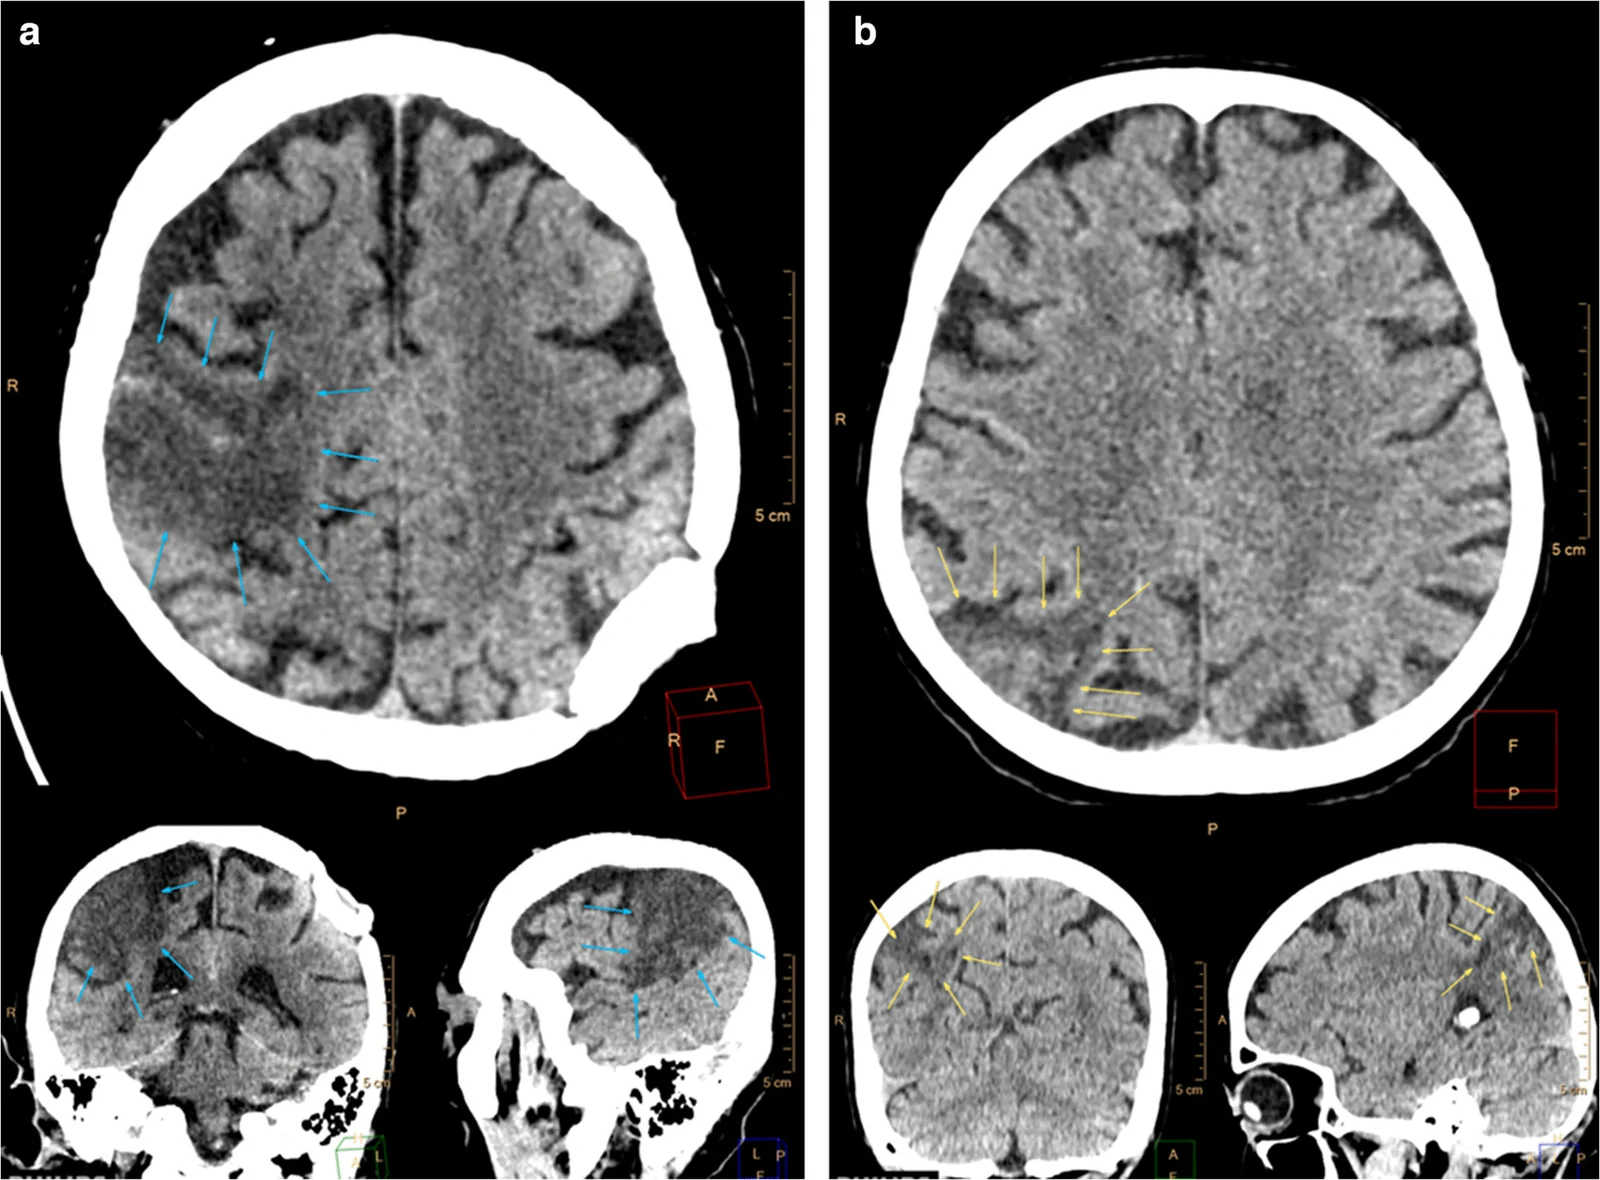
a, b Multiplanar CT reformats of a voluminous ill-defined cortical-subcortical hypodensity in the right frontal and parietal lobes (*blue arrows*), corresponding to a subacute middle cerebral artery territorial infarct of a patient with an exulcerated plaque and a carotid score of 4 (70% stenosis of the right internal carotid artery and no stenosis of the contralateral internal carotid artery). Left lateral craniectomy following surgical removal of an old meningioma; b ill-defined cortical-subcortical hypodensity in the right upper parietal lobe (*yellow arrows*), corresponding to a subacute triple watershed infarct of a patient with a carotid score of 1 (˃90% stenosis of the right internal carotid artery caused by a calcified plaque and 70% stenosis of the contralateral internal carotid artery)
